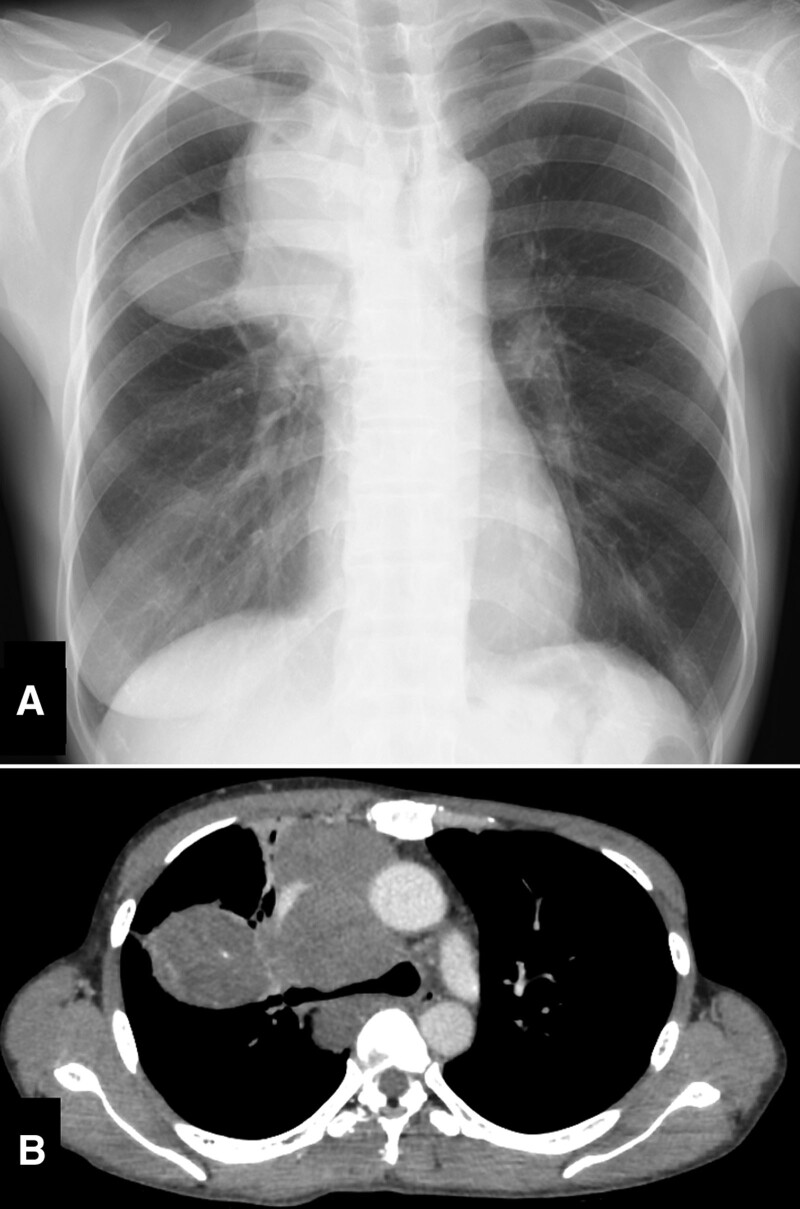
Pretreatment chest X-ray and contrast enhanced computed tomography of the tumor. (A) The chest X-ray showed a large mass in the right upper lobe without calcification. (B) The whole-body contrast enhanced (CE) computed tomography (CT) showed a large soft tissue mass 112 mm × 98 mm × 88 mm in size arising from the anterior mediastinum, expanding to the right lobe.
